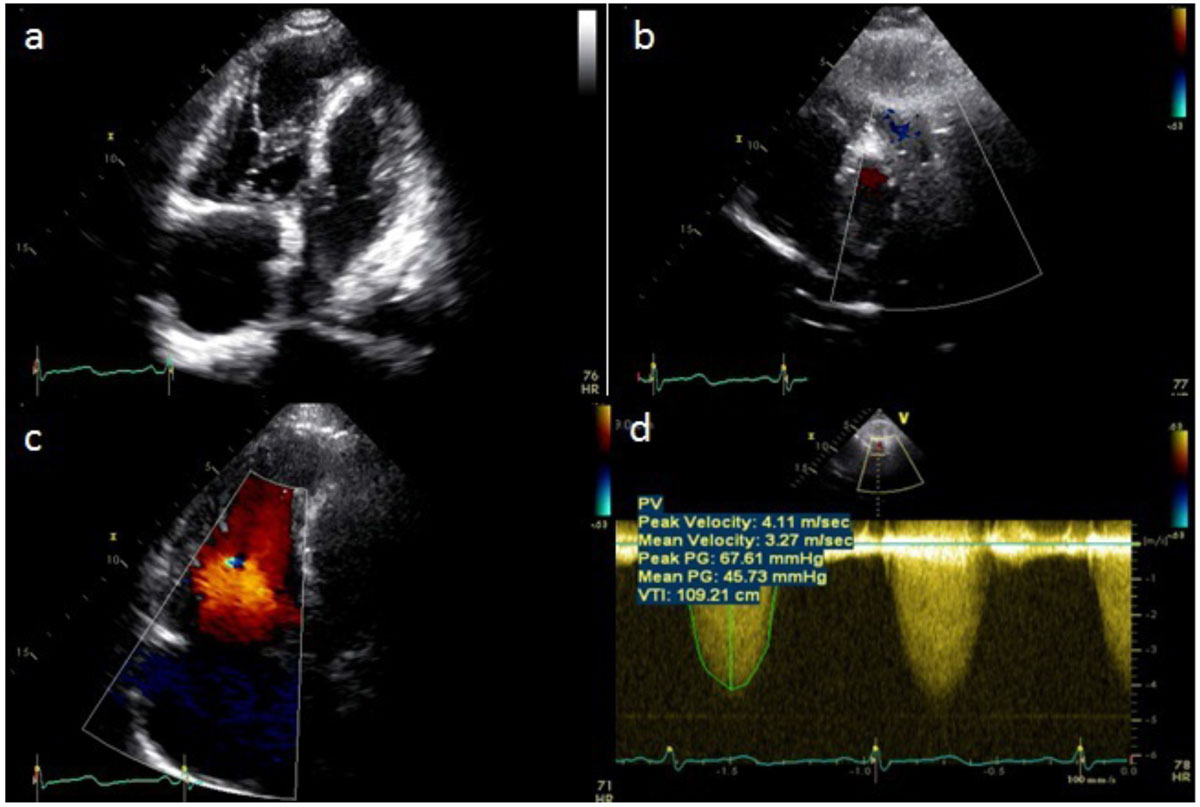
a. 4-chamber view, b. RVOT view, c. 4-chamber with color Doppler, d. CW Doppler at RVOT.
